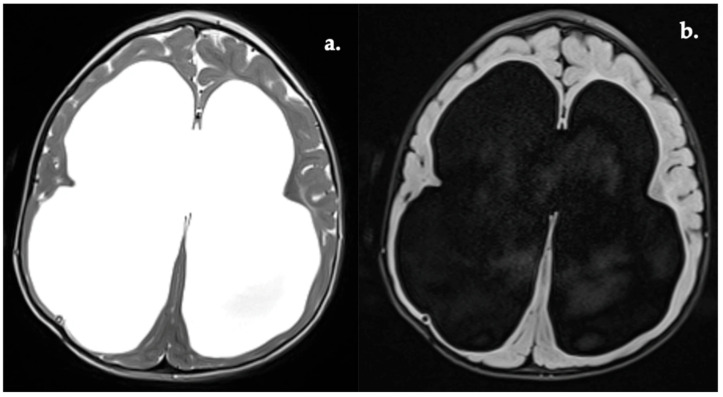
Ventriculomegaly (a) T2w image. (b) FLAIR image.
